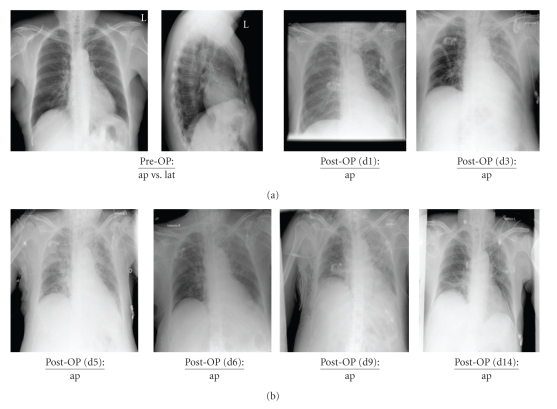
Chest X-ray diagnostic in the patient from preoperative (Pre-OP) until closely to the patient's discharge from hospital at day 14. Pre-OP: preoperative; d: day; ap: anterior-posterior; lat: lateral.
